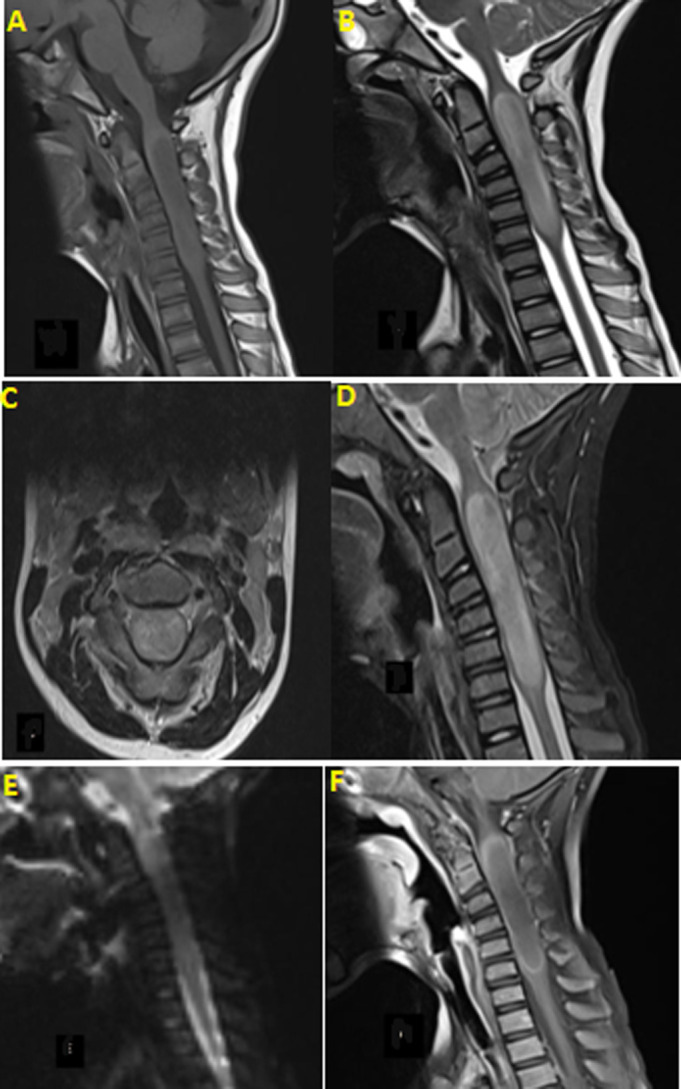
MRI images of the cervical spine; A) in sagittal T1; B) sagittal T2; C) axial T2; D) stir; E) diffusion; F) sagittal T1 after injection of gadolinium
